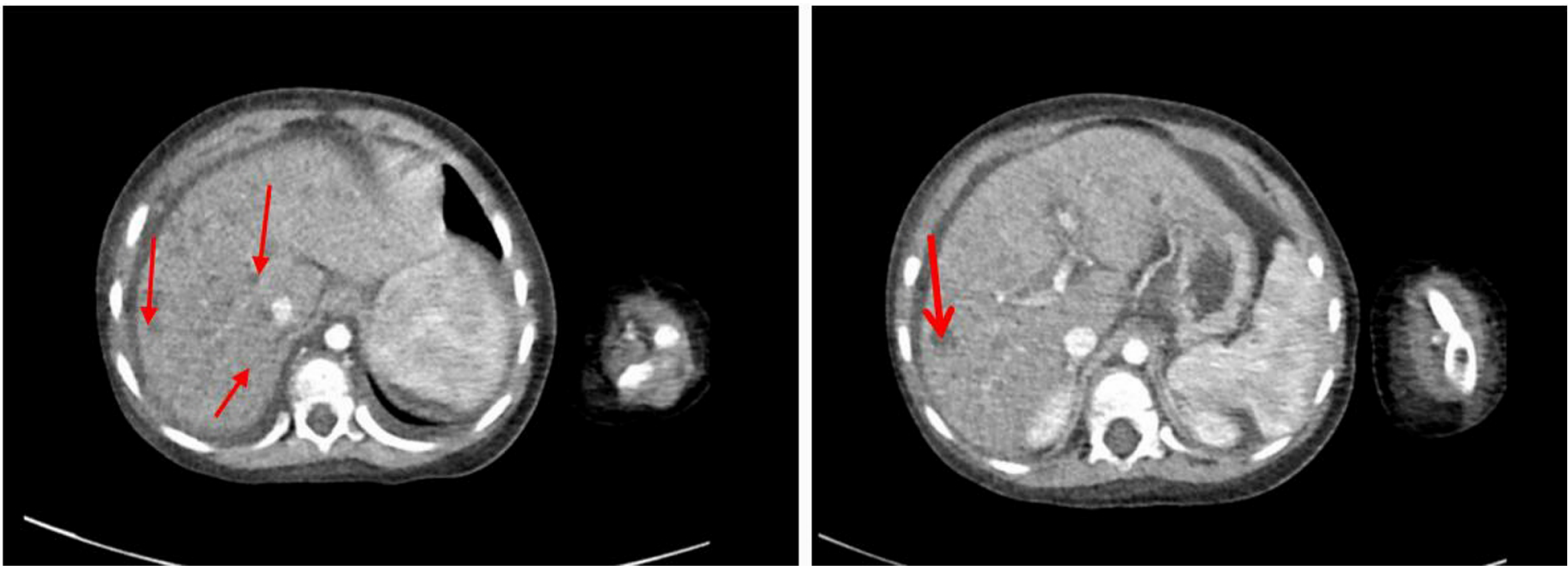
Computerized tomography (CT) of the liver. Multiple low-density foci were found in hepatic parenchyma.
